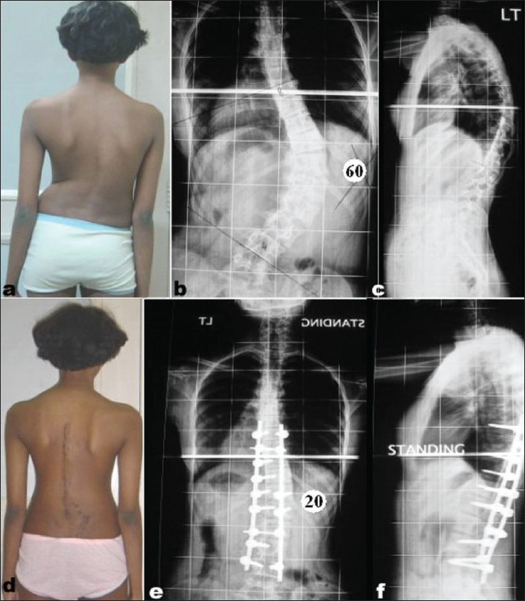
Clinical photograph (a) and anteroposterior (b)and lateral (c) radiograph showing typical King type IV curve with L4 tilted to the convexity of the curve with preoperative Cobb's angle of 60°. (ii) Follow up clinical picture (d) and radiographs, anteroposterior (e) and lateral (f) showing good correction. Cobb's angle has been reduced to 20°
